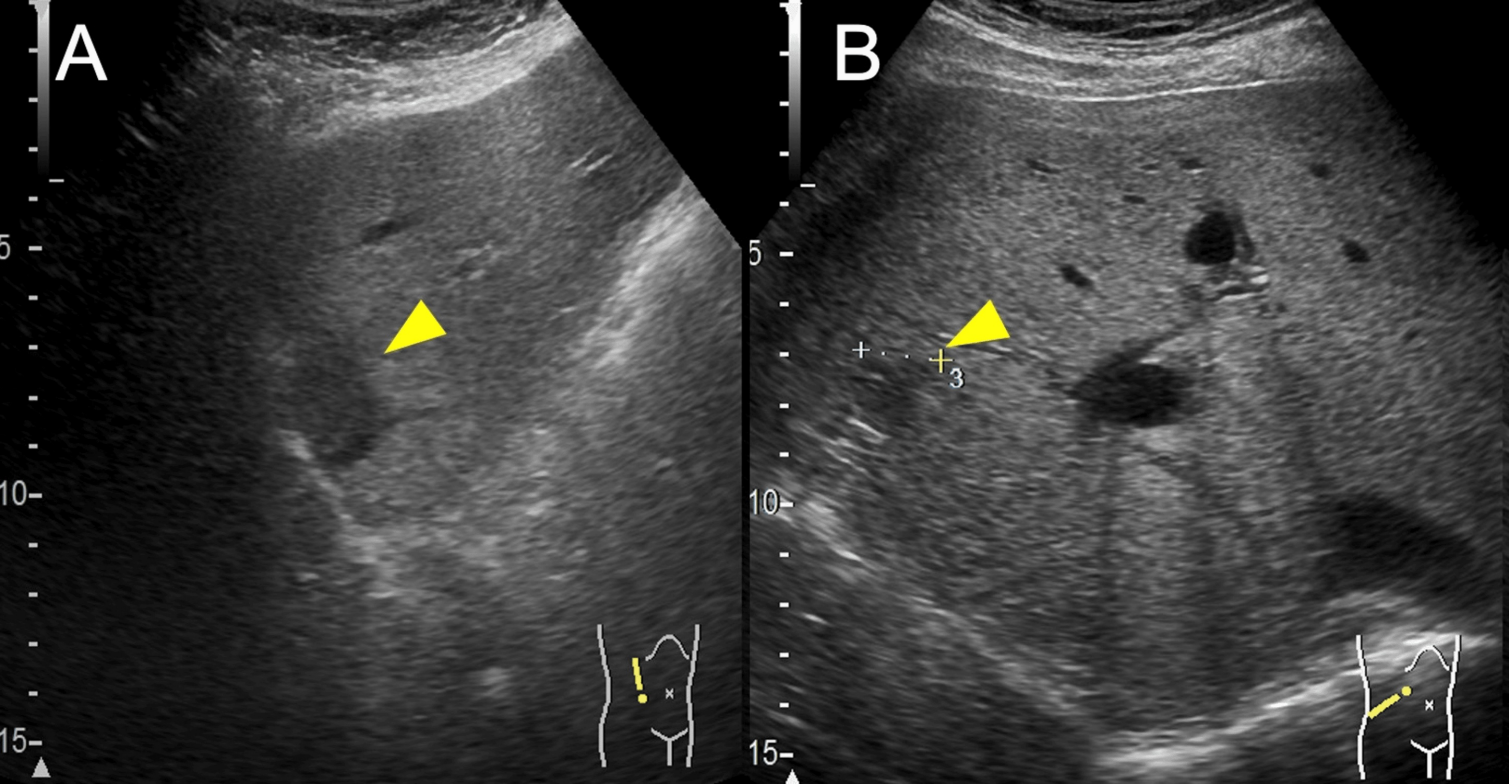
Ultrasonography image of the liver (A) Ultrasonography image demonstrating a hypoechoic mass in segment 2 of the liver (yellow arrowhead). (B) Ultrasonography image showing a hypoechoic mass in segment 6 of the liver (yellow arrowhead). Both lesions appear well-demarcated with homogeneous internal echoes, measuring approximately 2 cm in diameter. The surrounding liver parenchyma shows a coarse echotexture, consistent with chronic liver disease.
